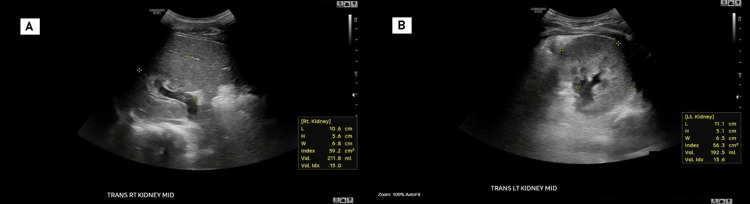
Renal ultrasound screening (A) Mild enlargement of the right kidney, with normal echotexture and cortical thickness. It measures 10.6 x 5.6 x 6.8 cm. No hydronephrosis, calculi, cysts, or solid masses are observed; (B) Mild enlargement of the left kidney, with normal echotexture and preserved cortical thickness. It measures 11.1 × 5.1 × 6.5 cm. Mild left-sided pelvicaliectasis is noted, which resolves following voiding, consistent with marked bladder distention. This finding excludes a pathological cause of pelvicaliectasis, such as hydronephrosis, a potential complication of Wilms tumor. No visible calculi are present. No cystic or solid masses are identified.
